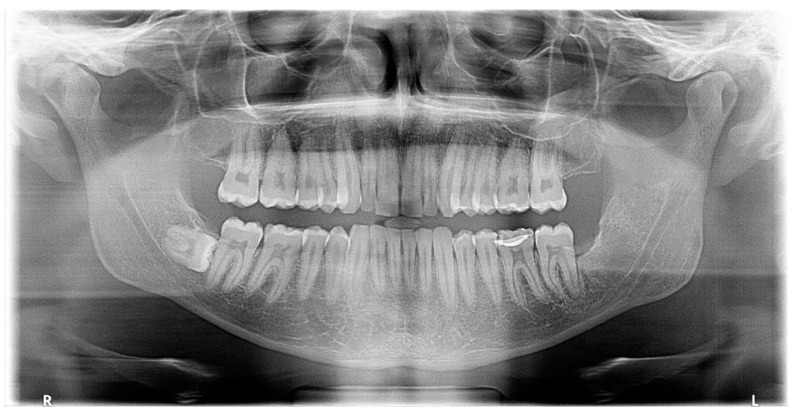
Panoramic X-ray of case #1 at one-year follow-up.
